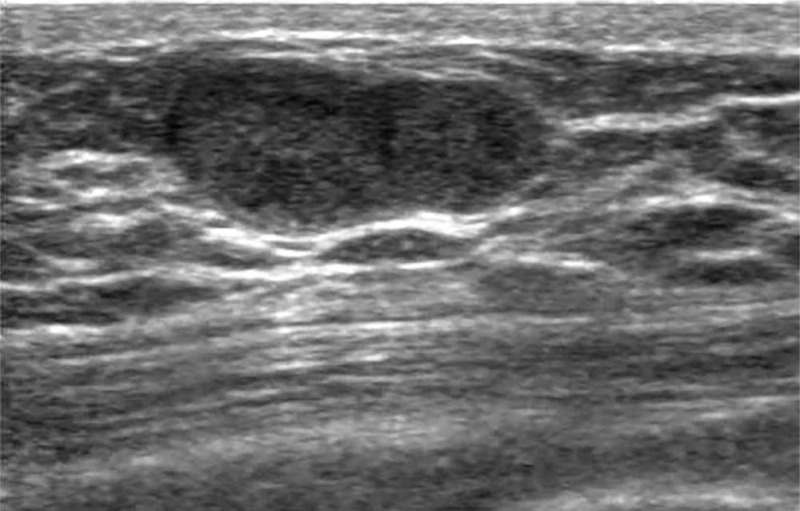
Ultrasonography demonstrated a regular shaped, well-defined hypoechoic mass in the subareolar region of the right breast.
